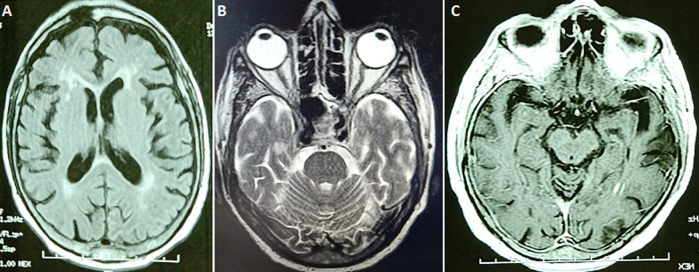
MRI images weighted in T1 (A), T2 (B) and flair (C) showing vascular leukopathy, with some calcifications in basal ganglia
